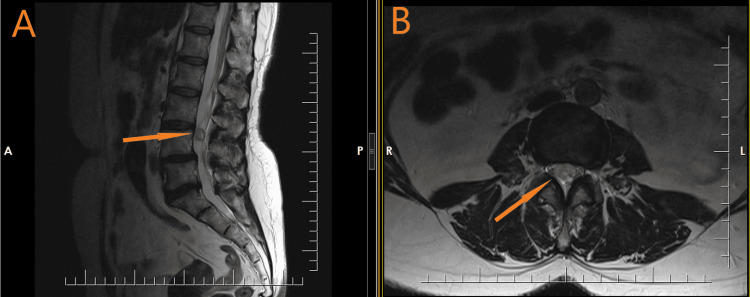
Sagittal (A) and axial (B) T2-weighted MRI showing an intradural mass at the level of the L4 vertebrae (arrows). MRI: magnetic resonance imaging
